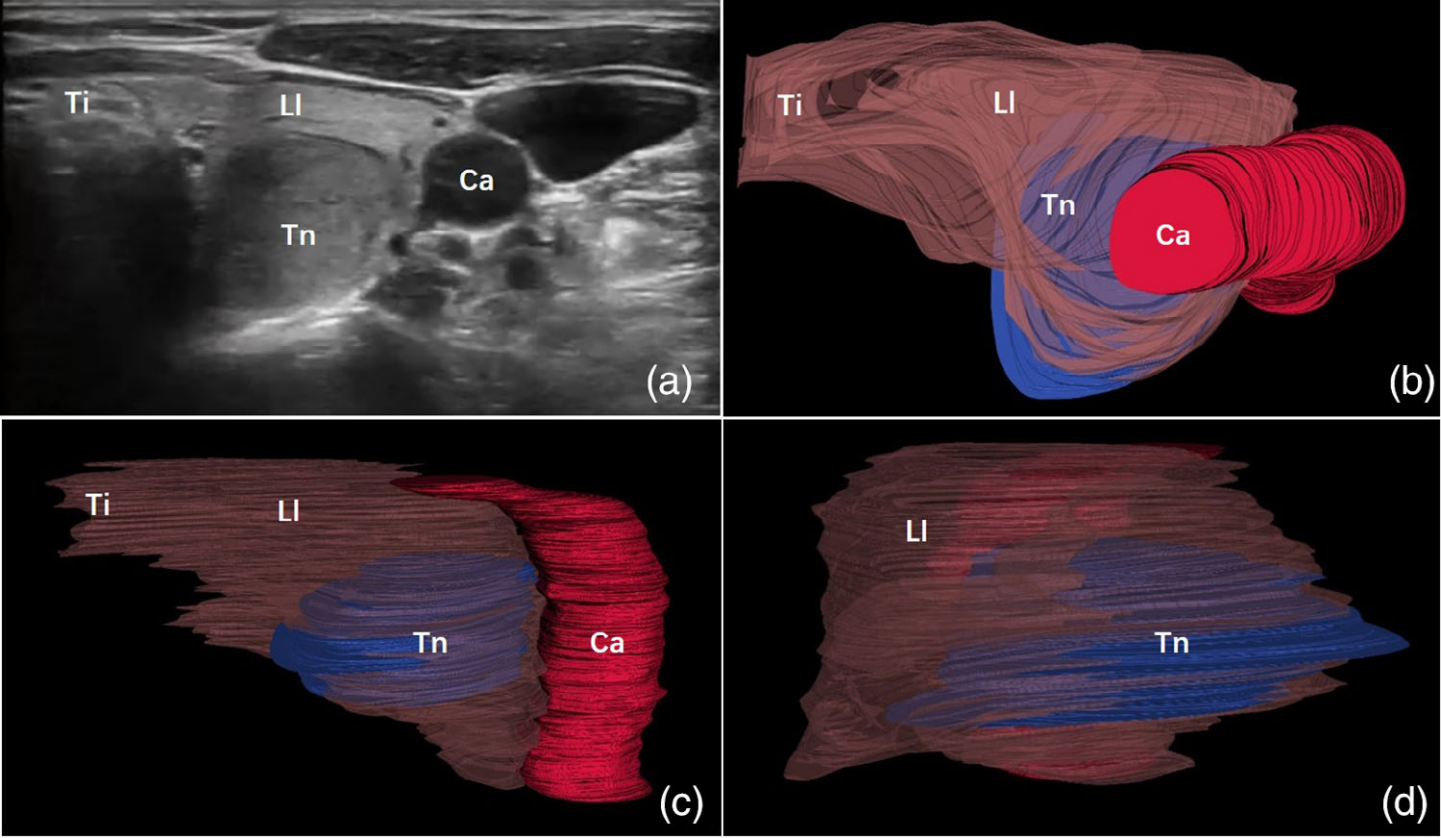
Images of a 34‐year‐old woman with a 38 mm thyroid nodule. The grayscale ultrasound image showed a thyroid nodule in the left thyroid lobe (a). The 3D reconstruction model could be rotated freely to get localization information of the nodule (b‐d). Images from the top view (b), front view (c), and side view (d) of the 3D reconstruction model were available. The nodule was shown in blue, the thyroid lobe was shown in brown and the carotid artery was shown in red. Ca, carotid artery; Ll, left lobe of thyroid gland; Ti, thyroid isthmus; Tn, thyroid nodule.
